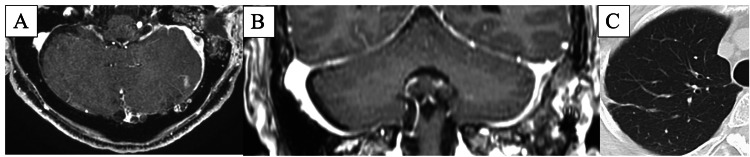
Follow-up imaging after treatment (A) Magnetic resonance imaging performed two months after treatment showing resolution of the subdural empyema. (B and C) Magnetic resonance imaging performed one year after treatment showing a reduction in the size of the thrombosis in sigmoid sinus (B) and the absence of septic pulmonary emboli (C).
